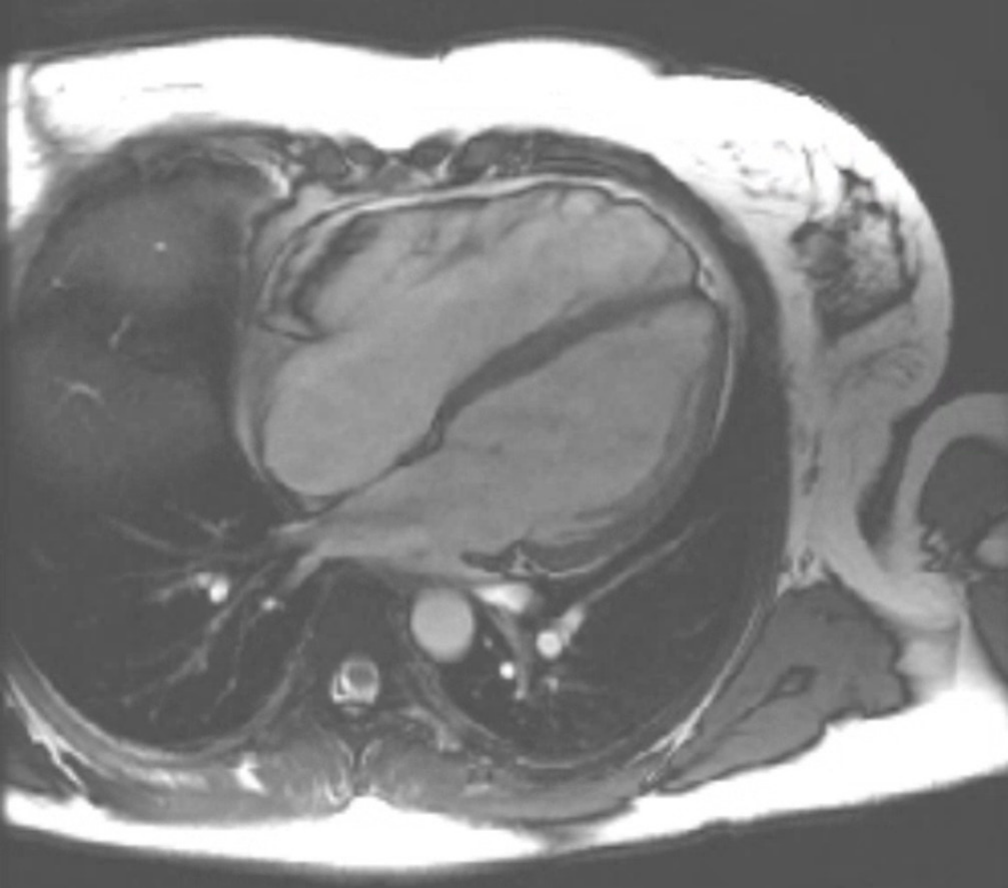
Four-chamber MRI view of patient C. Distinct tubular dilatation of the right ventricle (RV) with dyskinesia, impaired RV ejection fraction (34%) and elevated RV end-diastolic volume index (132.4 mL/min). Also oedema and late enhancement in the wall of the right ventricle.
